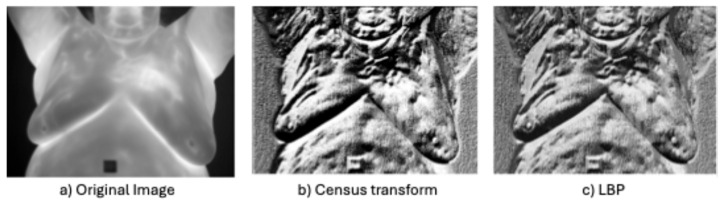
Comparison of a grayscale image of patient #180 (abnormal) from the DMR-IR dataset [39] with binary encoded CT and LBP setting R = 2 and P = 8.
